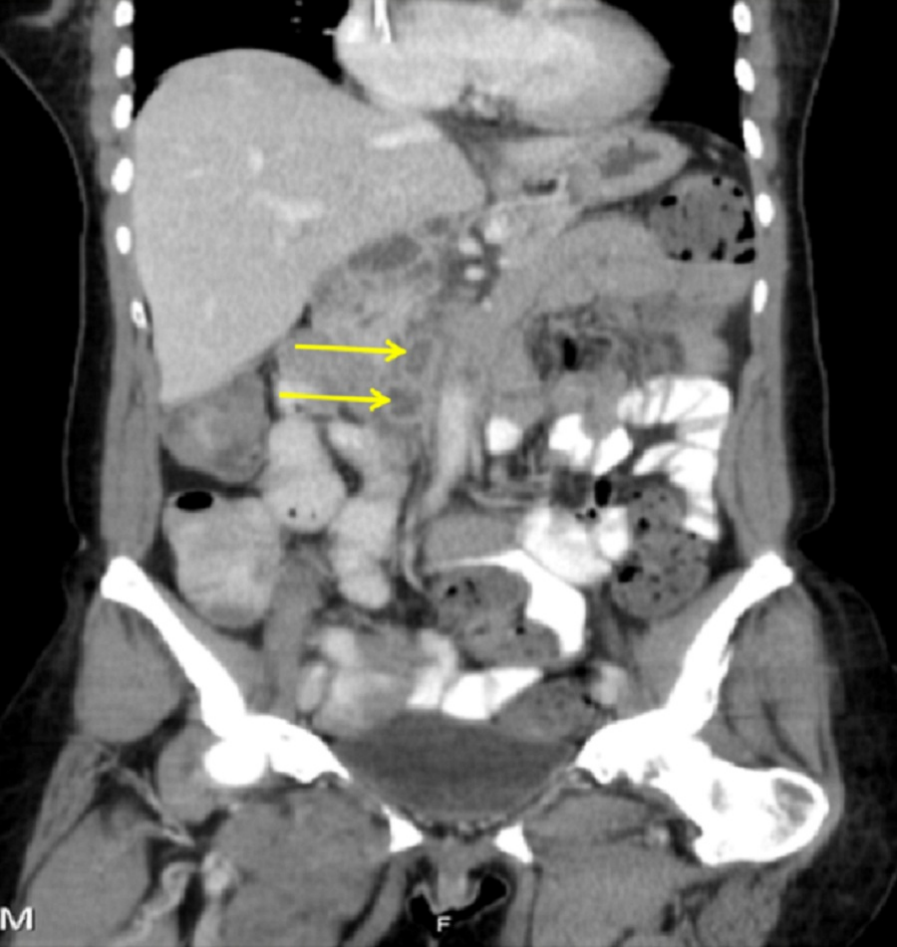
CT abdomen and pelvis (transverse section) CT abdomen and pelvis showing left para-aortic and aortocaval lymph node enlargement, measuring 1.2 and 1.4 centimeters in diameter, respectively. Mesenteric or pelvic lymphadenopathy was not present.
